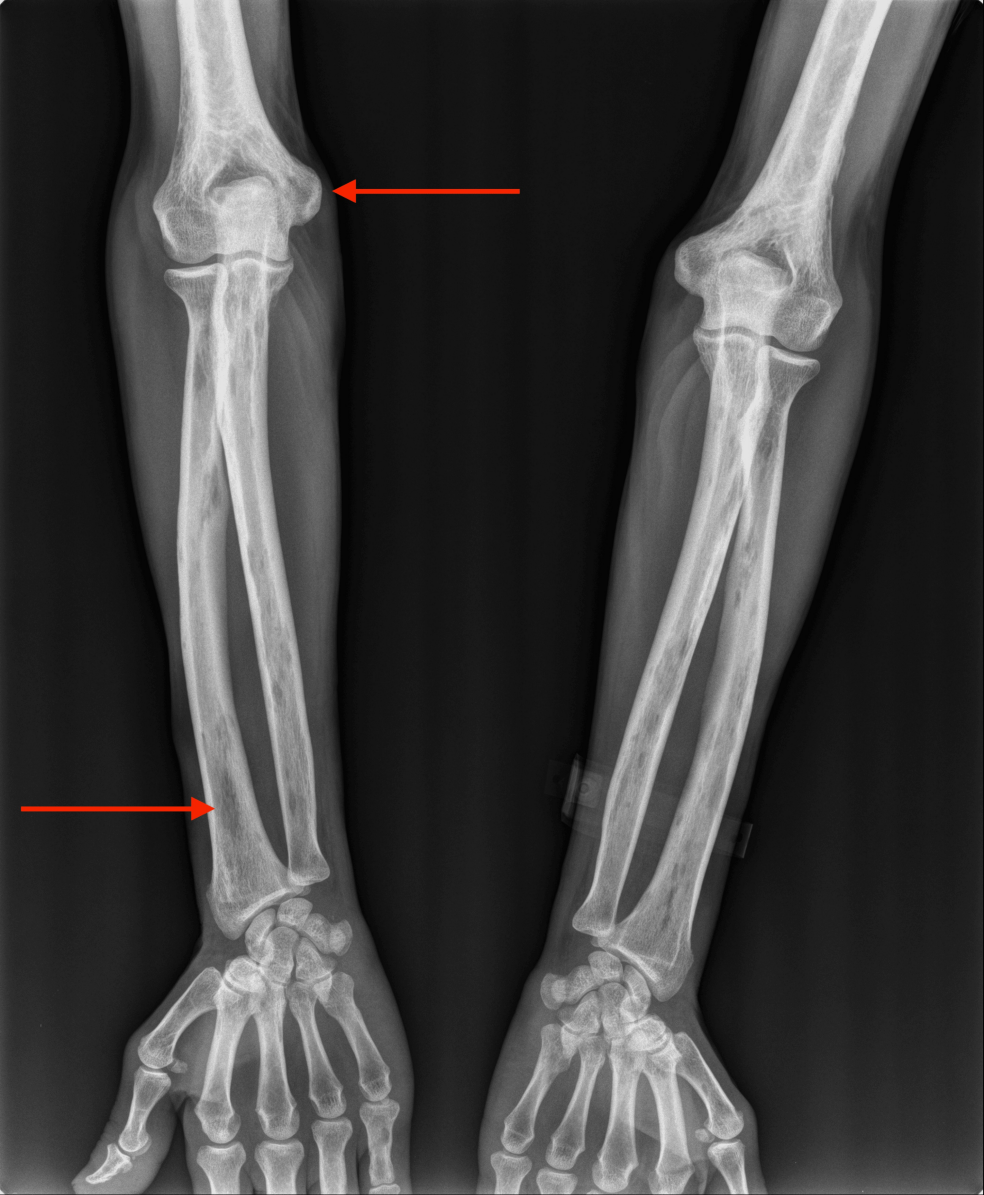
X-ray of the forearm Osteolytic lesions present in the humerus, ulna, and radius (red arrows)
